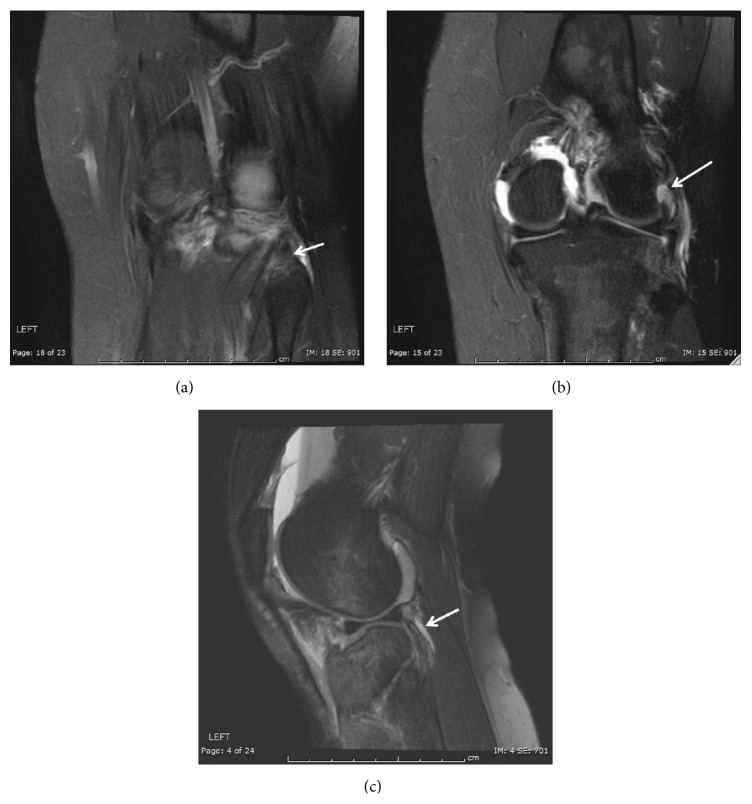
MRI of left knee showing popliteofibular ligament tear and edema in fibular styloid (arrow, (a)), attenuated LCL (arrow, (b)), and popliteus tendon signal abnormality suggesting interstitial tearing (arrow, (c)).
